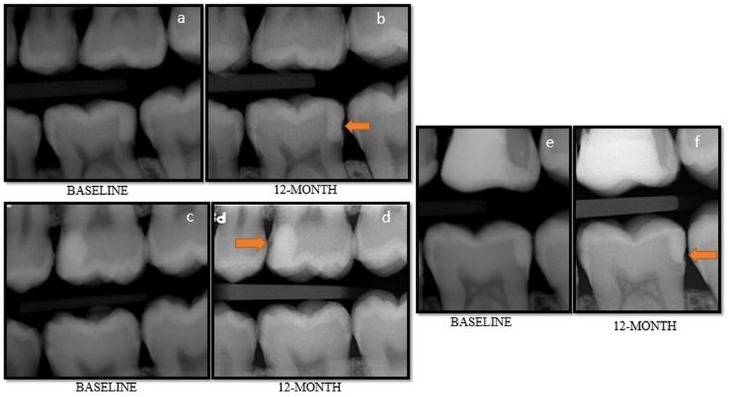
Glass ionomer material loss that could be radiographically seen in the proximal area as a result of dissolution
